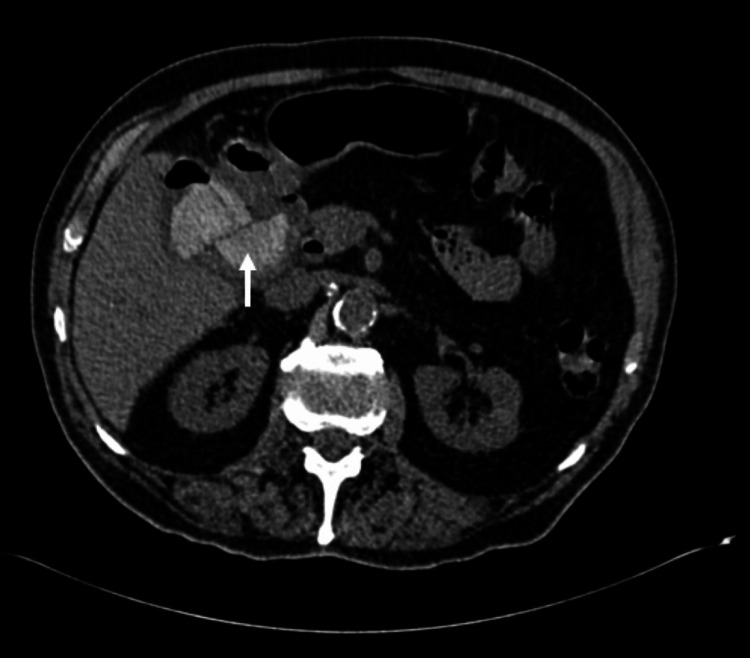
Axial CT non-contrast gallstone within the lumen of the duodenum (white arrow).
